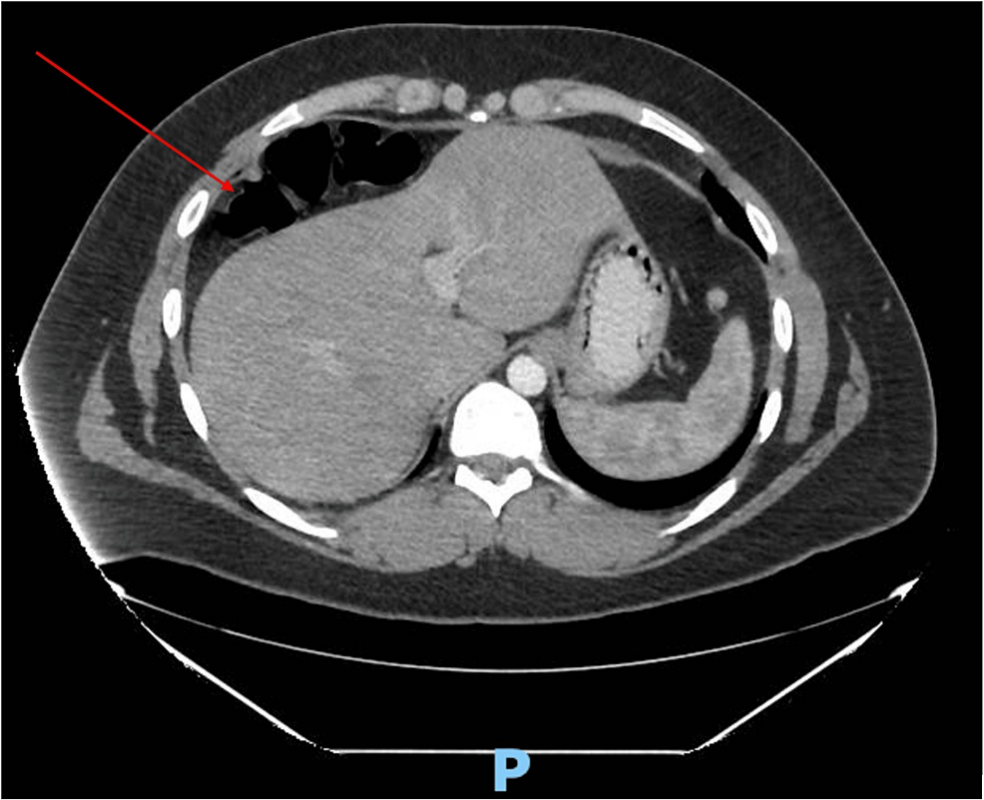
Axial CT of Right Colonic Interposition (Red Arrow)
